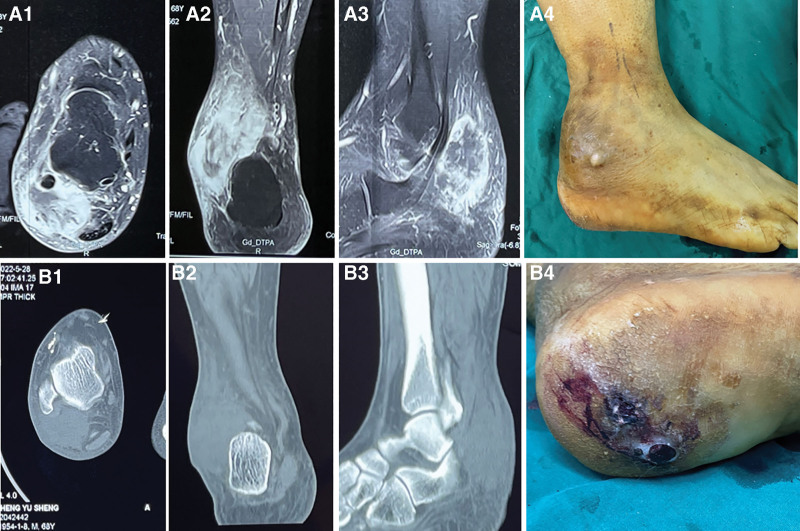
Displays the CT and MRI examinations as well as the appearance of the lesions. A1, A2, and A3 show the transverse, coronal, and sagittal MRI views of the ankle part tumor, respectively. B1, B2, and B3 show the transverse, coronal, and sagittal MRI views of the plantar part tumor, respectively. A4 and A5 depict the appearance of the tumors in the ankle and plantar regions, respectively.
